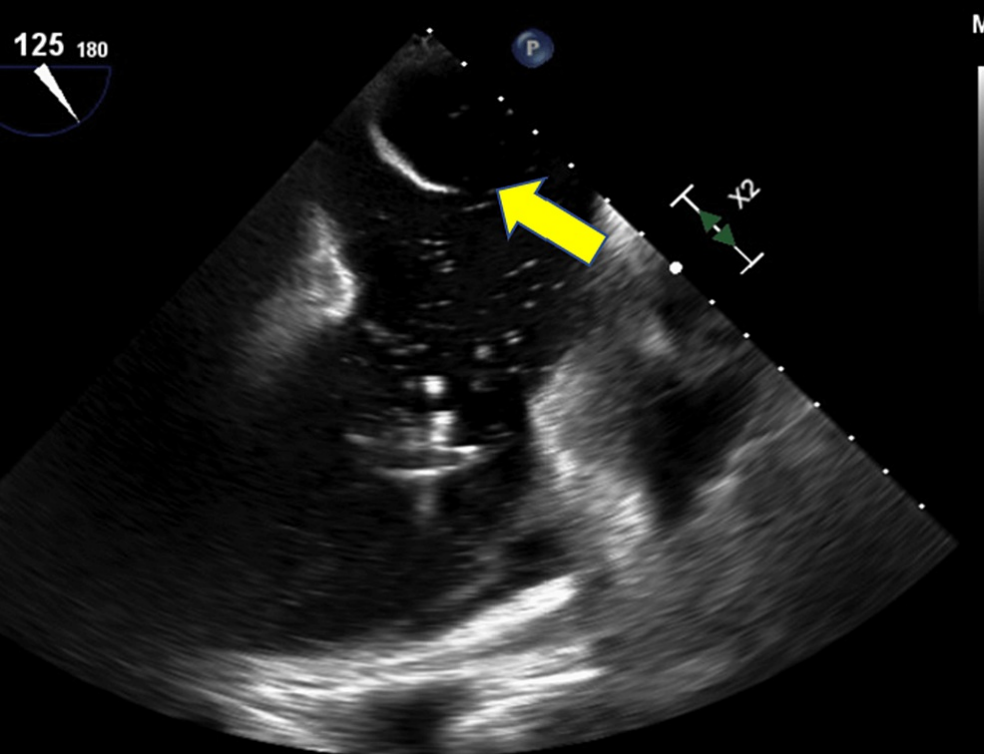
TEE showing a possible atrial septal defect. The yellow arrow indicates what appears to be a communication between the left atrium and the right atrium concerning for an atrial septal defect. TEE, transesophageal echocardiogram
